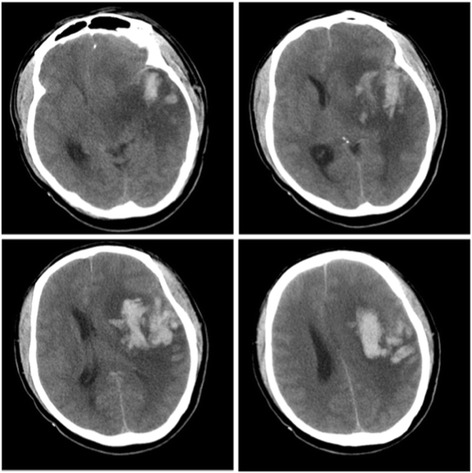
Brain computed tomography images showing left temporal lobe insular lobe infarction and haemorrhagic transformation
